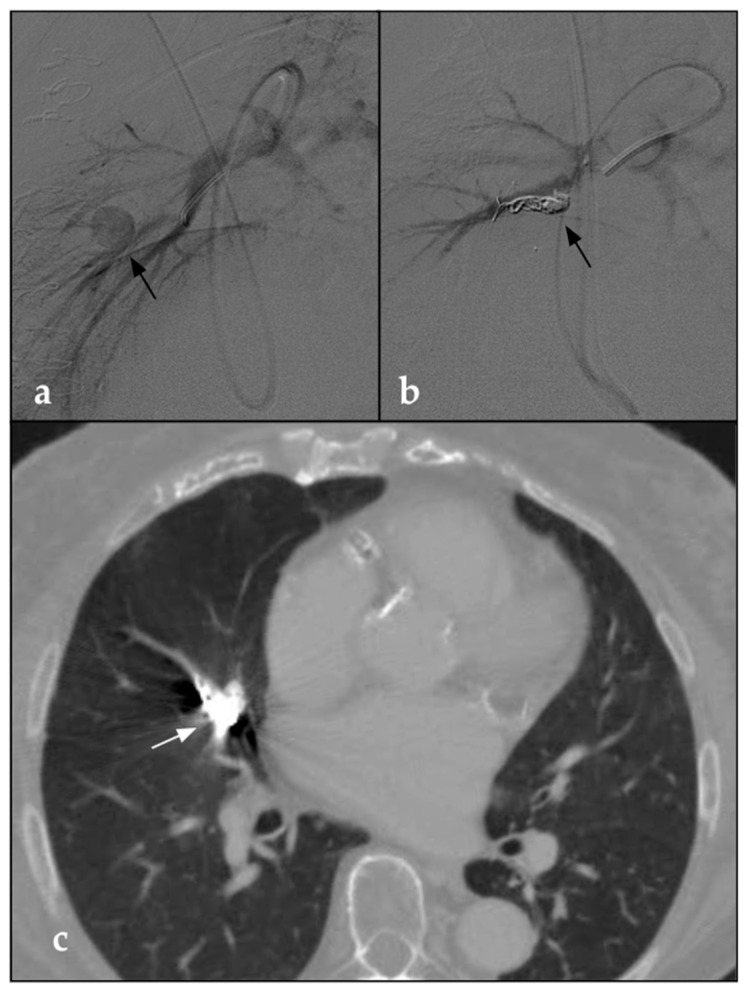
(a) DSA parasagittal image shows a 5 F catheter with the distal end at the level of the origin pulmonary artery tributary of the middle right lobe. The arteriographic study demonstrates a voluminous round-shaped pseudoaneurysm in the middle portion of the tributary branch of the middle right lobe (black arrow). (b) Post-procedural parasagittal DSA shows the complete embolization of the pseudoaneurysm through the deployment of detachable coils (black arrow). (c) Post-procedural CTA on the axial plane taken 1 month after the procedure confirms the complete embolization of the vascular lesion in the presence of coils (white arrow). No signs of pulmonary infarction are detectable.
